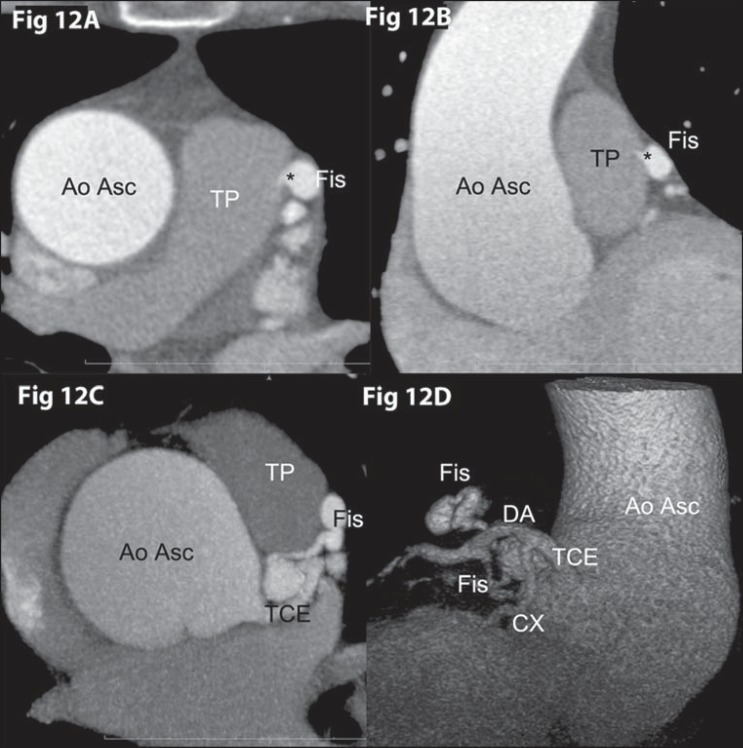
Coronary fistula*. Coronary computed tomography angiography
